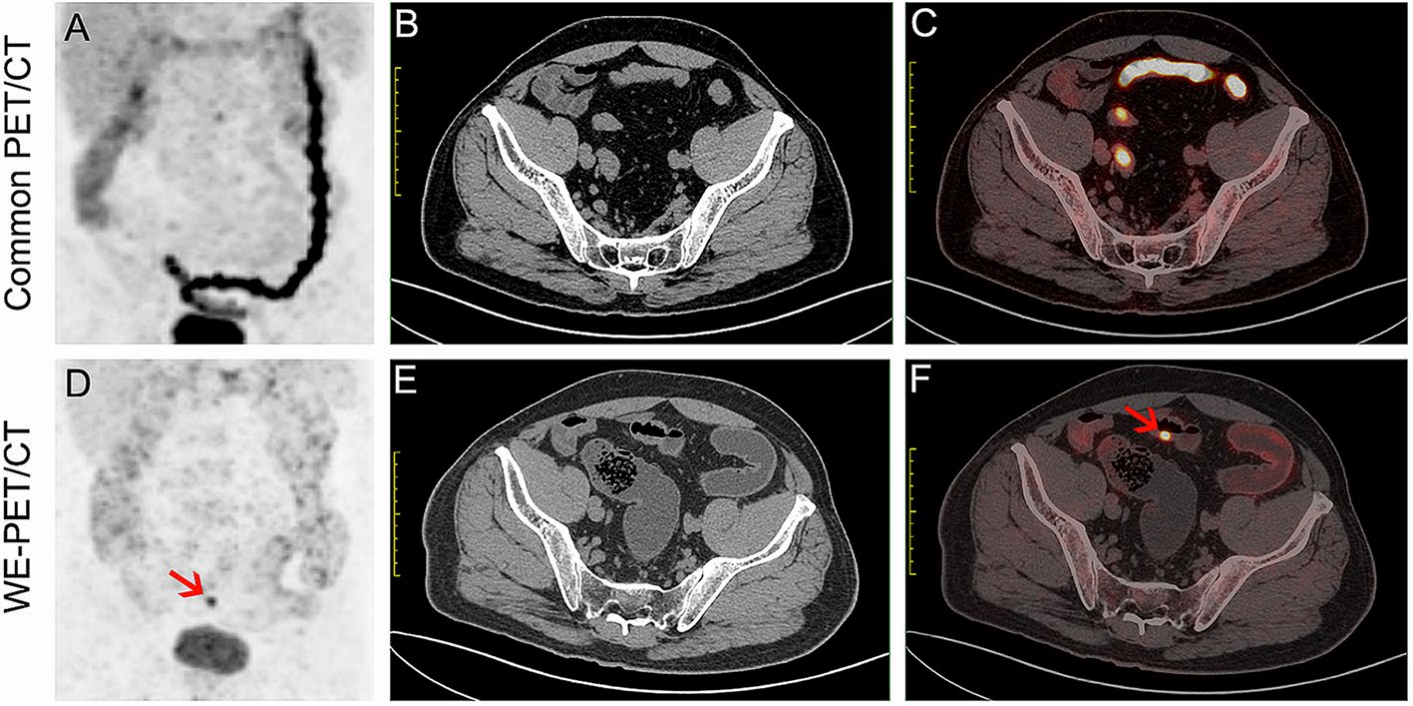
A small lesion hidden in diffuse ^18^F-FDG uptake. Strong diffuse ^18^F-FDG uptake in the colorectum with a collapsed bowel is shown on the CT scan (A-C). After water enema, diffuse ^18^F-FDG uptake disappeared except for a focal ^18^F-FDG-avid lesion in the sigmoid with localized wall thickening (D-F, red arrow)
